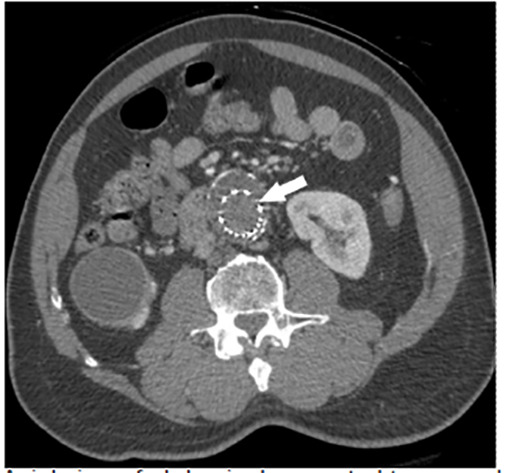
Axial view of abdominal computed tomography angiography demonstrating absence of contrast within the aortic endograft, an indication of occlusion (arrow).
